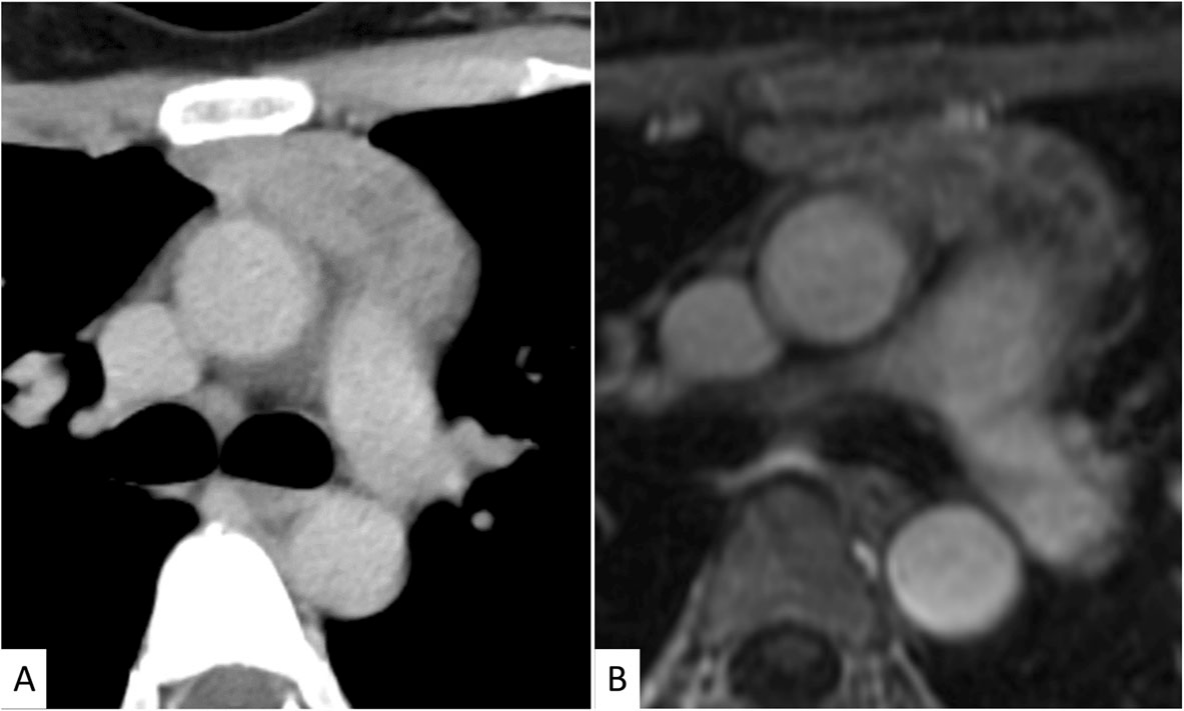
a Computed tomographic scan of the chest showing a well-circumscribed mass with a heterogenous concentration measuring 49 × 22 mm in the anterior mediastinum. b Out-of-phase dynamic magnetic resonance imaging of the mediastinum showing the multilocular mass without invasion to the surrounding parenchyma
